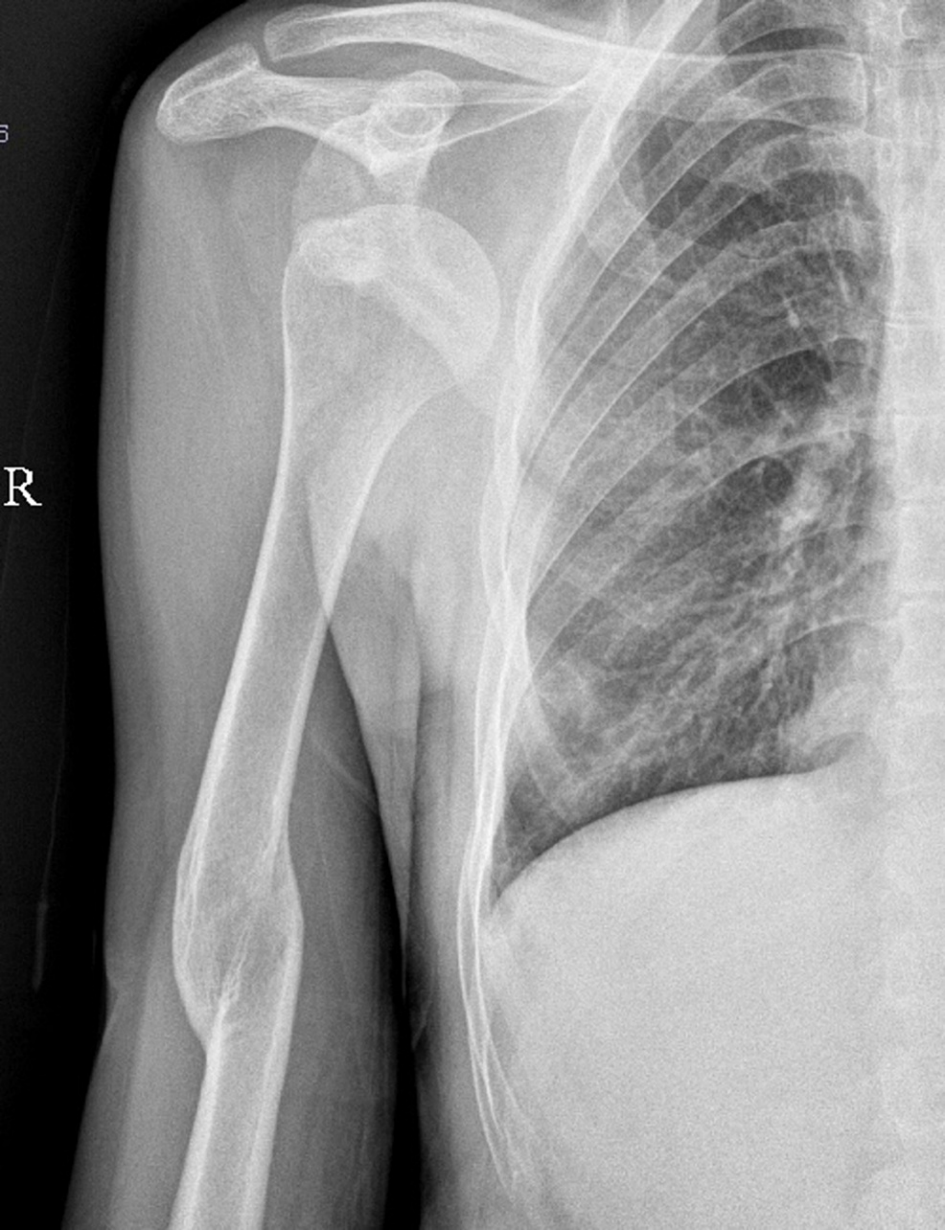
Pre-operative X-ray showing a subcoracoid subtype of anterior shoulder dislocation.
